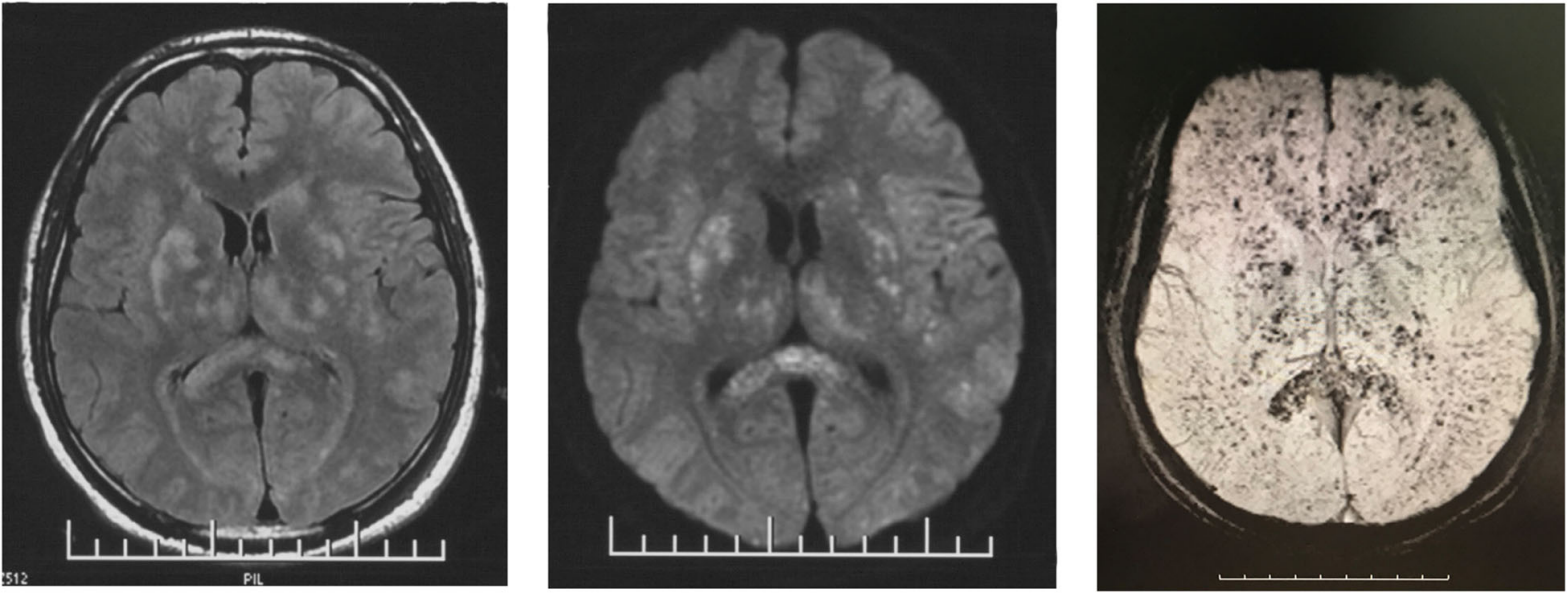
MRI scans obtained 3 days after the accident (FLAIR, DWI, SWI sequence in order)
